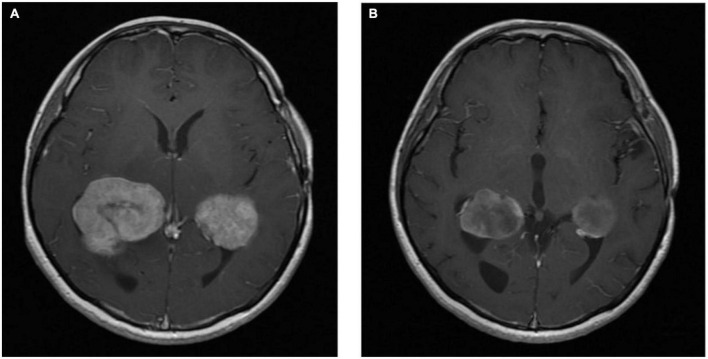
Axial T1 post-contrast MRI images *Rosai-Dorfman* disease pre-BRAF therapy (A) and 3 months post-BRAF therapy (B).
